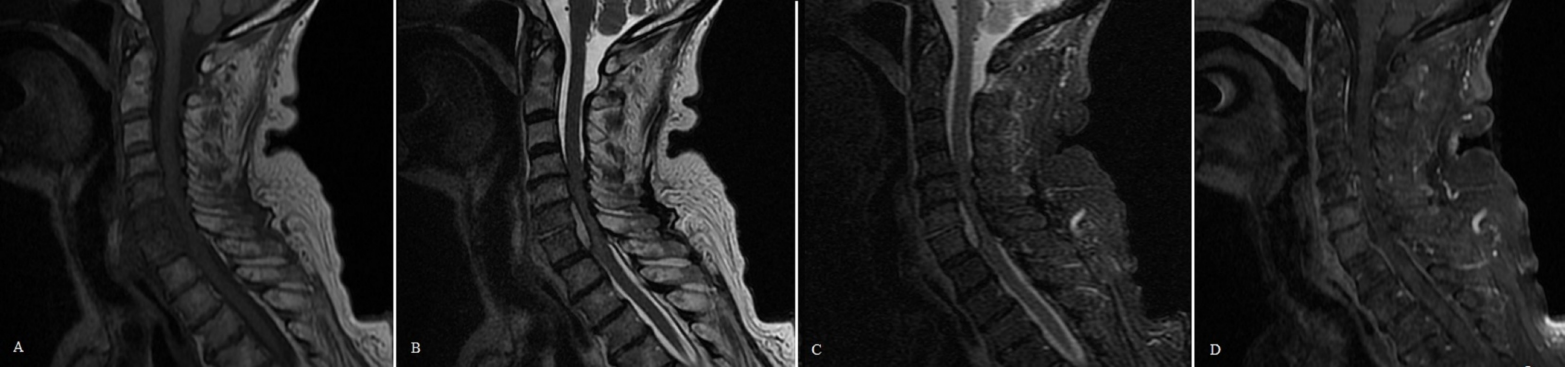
Sagittal MRI of Cervical Spine at Presentation A) T1-weighted images showing isointense epidural collection at the level of C6/7 and hypointense vertebral bodies of C6 and C7. B) T2-weighted images showing a hypointense epidural collection at the level of C6/7 concerning for hematoma with a slight hyperintensity of C6 and the C6 vertebral bodies. C) Short T1 inversion recovery (STIR) sequences images showing prevertebral edema and disruption of the anterior longitudinal ligament at C6/7 and slight hyperintensity of C6/7 disc space and C6 and C7 vertebral bodies likely representing bone marrow edema concerning for underlying injury though no discrete fracture line is noted. D) T1-weighted image with contrast; no significant ring enhancement of the collection is noted.
